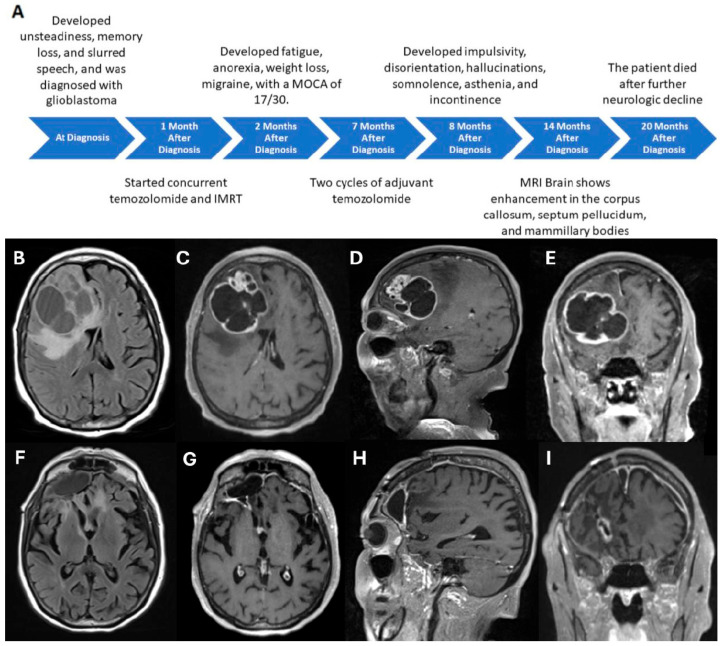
Chronology of clinical progression and MRI findings. (A) Timeline depicts the patient’s progressive clinical course (A). A multi-cystic enhancing glioblastoma with surrounding vasogenic edema in the right frontal lobe is shown in T2/FLAIR-weighted axial sequence (B) as well as post-gadolinium T1-weighted sequences in axial (C), sagittal (D), and coronal (E) views. Stable resection cavity and slight thickening of the septum pellucidum is seen in T2/FLAIR-weighted axial sequence (F) as well as post-gadolinium T1-weighted sequences in axial (G), sagittal (H), and coronal (I) views. IMRT: intensity-modulated radiotherapy, MOCA: Montreal Cognitive Assessment.
